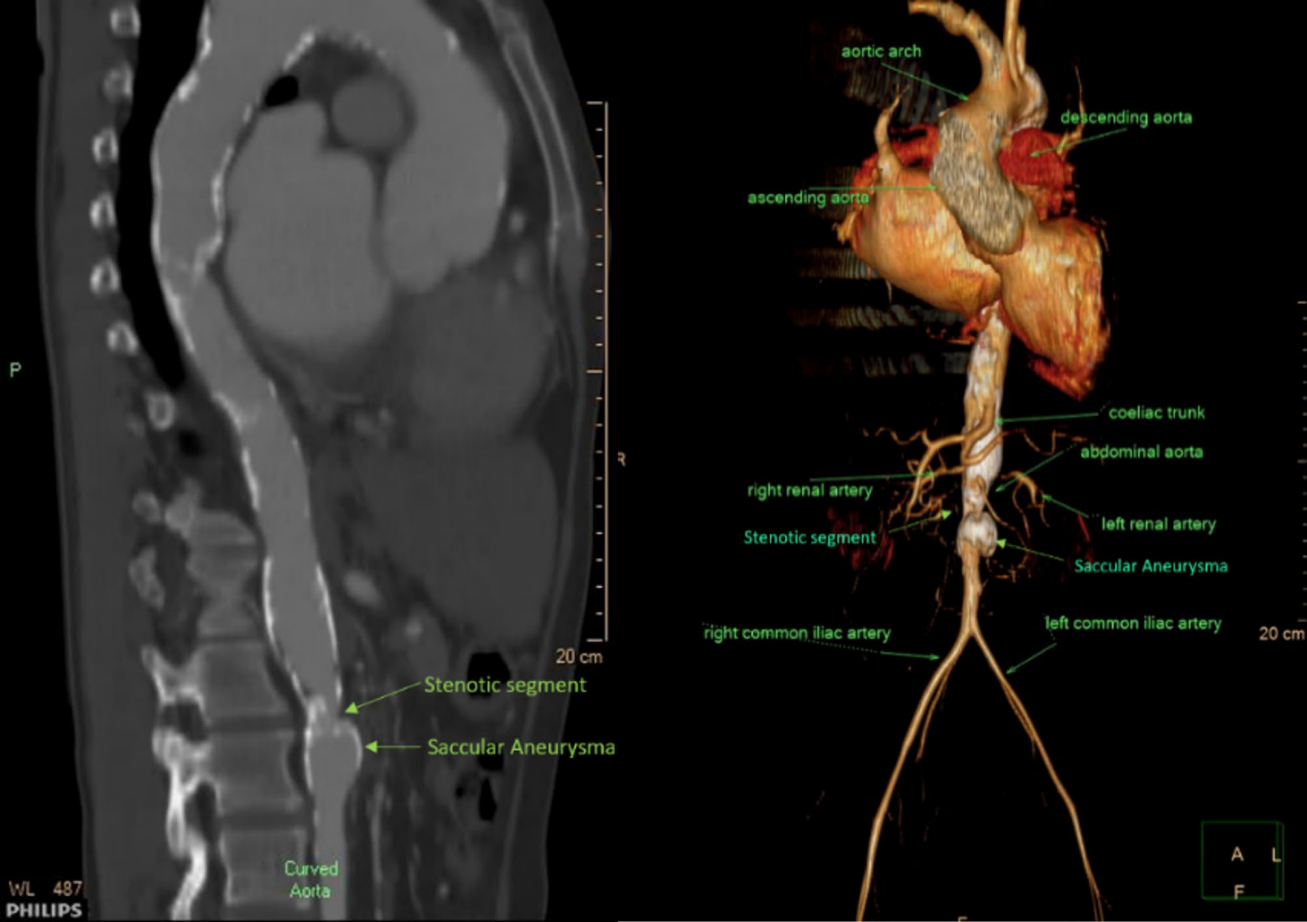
Computed tomography angiography (CTA) revealing significant stenosis in the infrarenal abdominal aorta accompanied by a saccular aortic aneurysm
